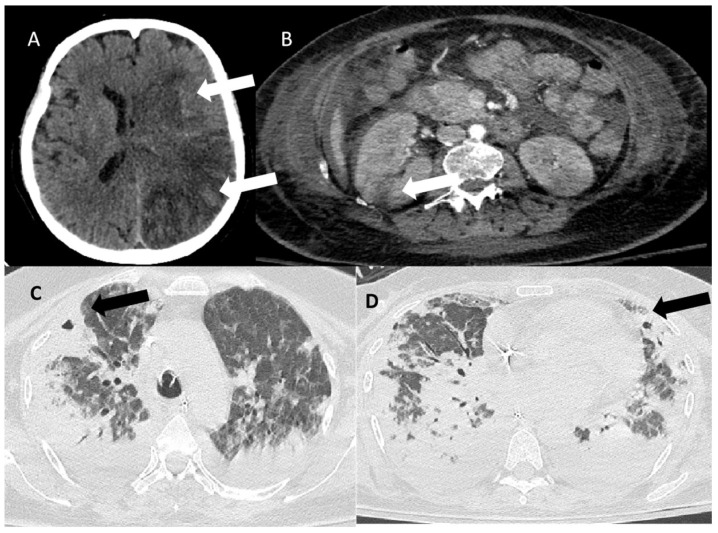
Computed tomography findings. (A) Brain CT showing multiple ischemic lesions located in the left frontotemporolateral region (white arrow) and in the right frontal lobe. Effacement of the left lateral ventricle and displacement of the midline structures by 0.5 cm to the right. Trace of subarachnoid hemorrhage (white arrow). (B) Ischemic infarct at the periphery of the right kidney (right arrow). (C) Cavitary lesions in the right upper and (D) left lower lobe (black arrows).
